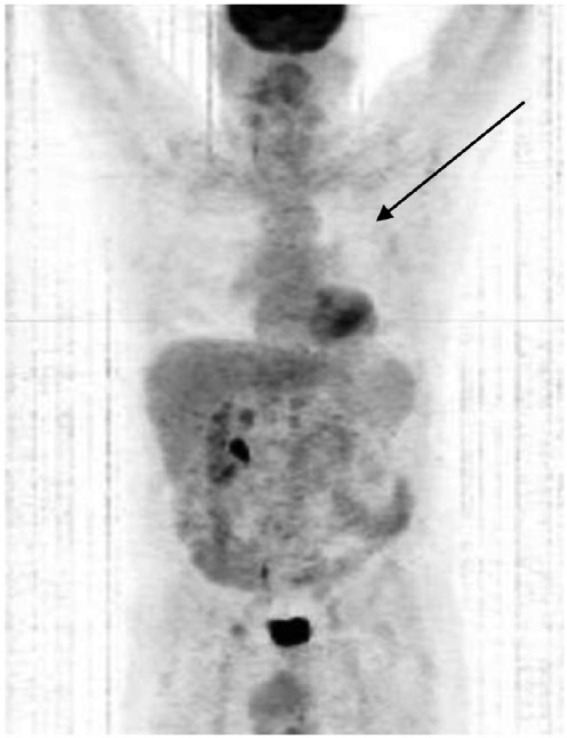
PET/CT scan, August 2014.
